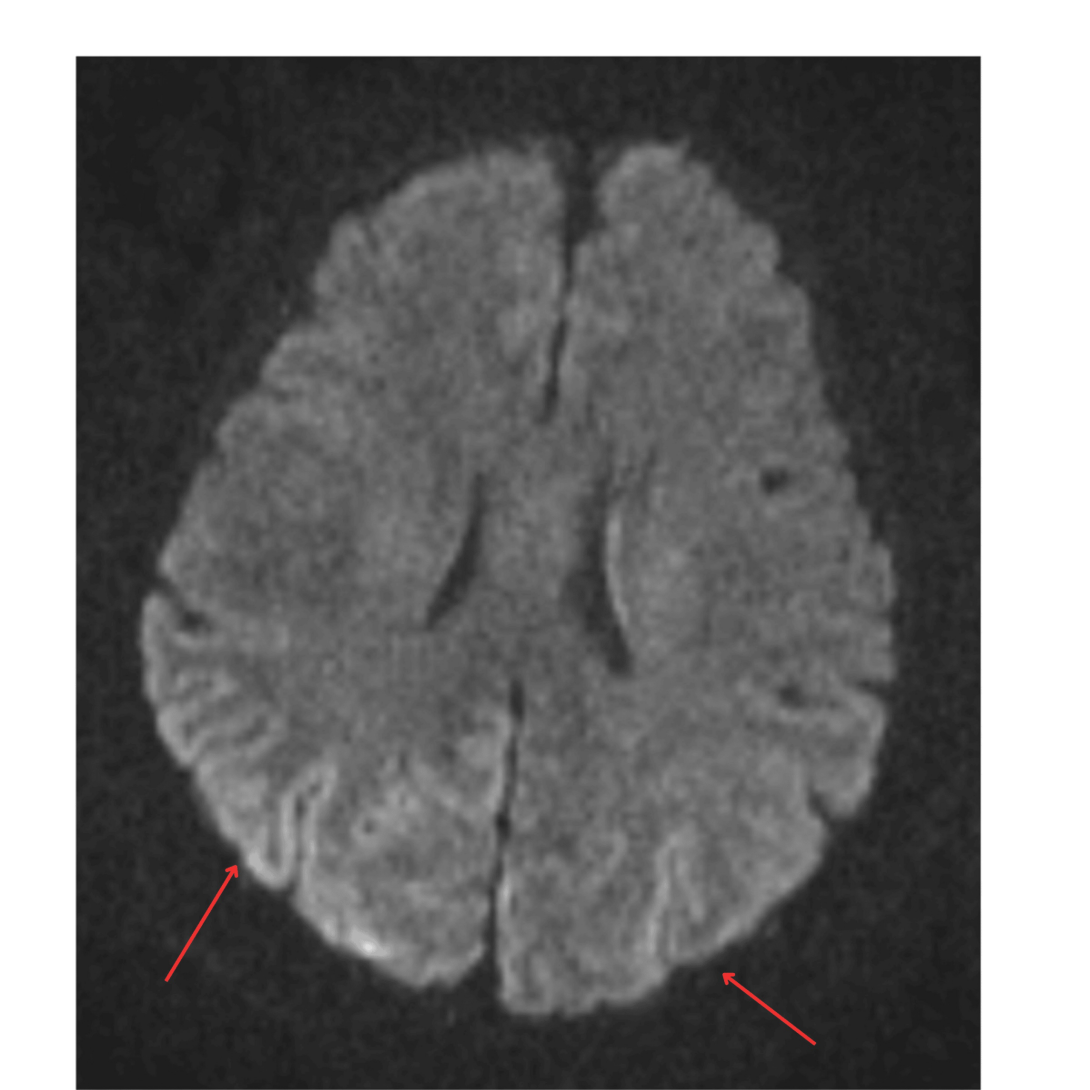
MRI brain, diffusion-weighted imaging MRI: Magnetic resonance imaging Cortical diffusion restriction of both cerebral hemispheres posterolaterally indicated by the two bilateral arrows. Limited study due to motion artifact
